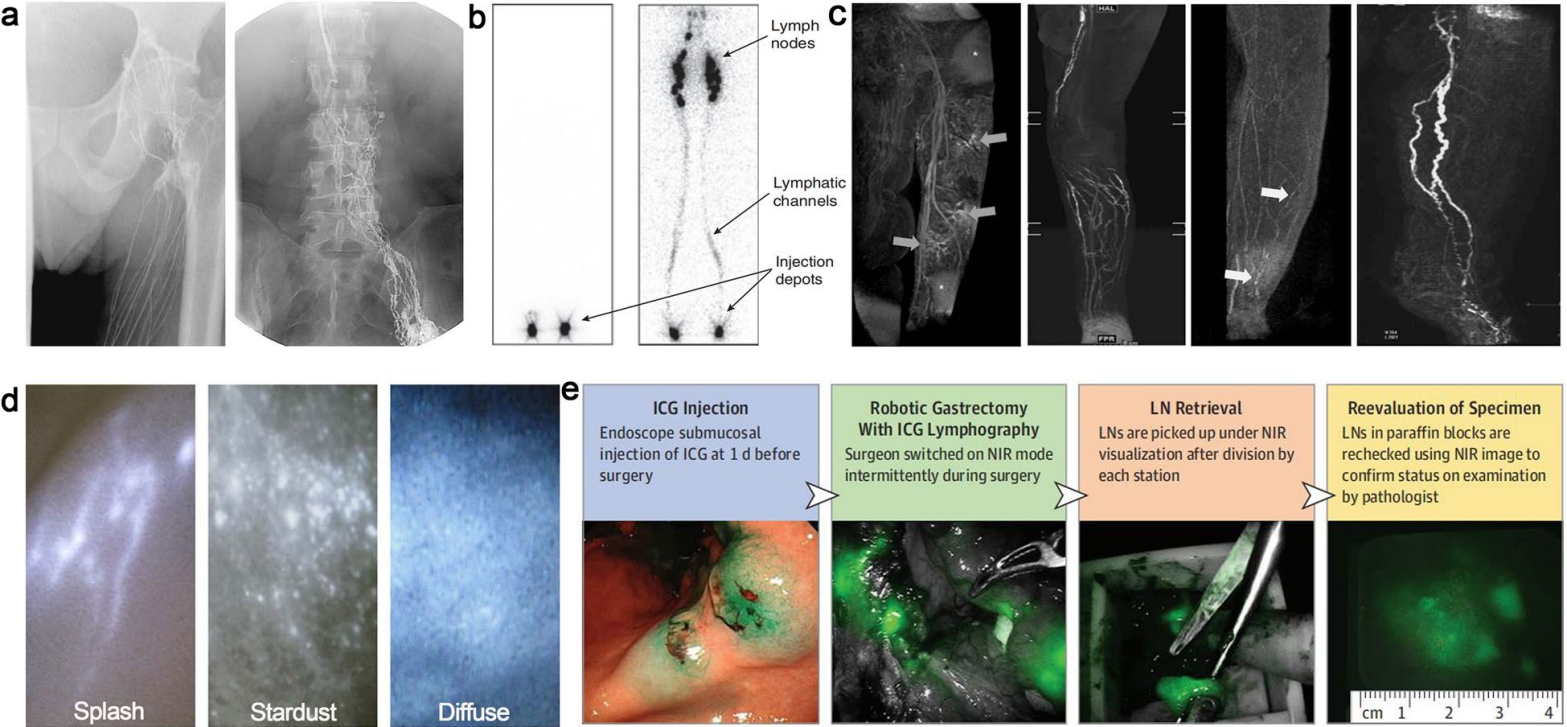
Current clinical imaging modalities of lymphatic system. a Typical radiographic image of the inguinal region following iodide angiography. Reproduced with permission [25]. Copyright 2019, Lippincott Williams and Wilkins. b Systemic lymphoscintigram after subcutaneous injection of radioactive tracers in the fee. Reprint with permission from [26]. Copyright 2010, Wiley–VCH Verlag GmbH & Co. KGaA, Weinheim. c Different MRL patterns of lymphatic drainage in lymphoedematous limbs. Reproduced with permission from [27]. Copyright 2015, Wiley–VCH Verlag GmbH & Co. KGaA, Weinheim. d Characteristic of indocyanine green (ICG) lymphography in different lymphedema stages, Reproduced with permission from [28]. Copyright 2014, SAGE Publications Inc. e Procedure to defining fluorescent LNs in robotic gastrectomy. Reproduced with permission from [29]. Copyright 2018, American Medical Association
